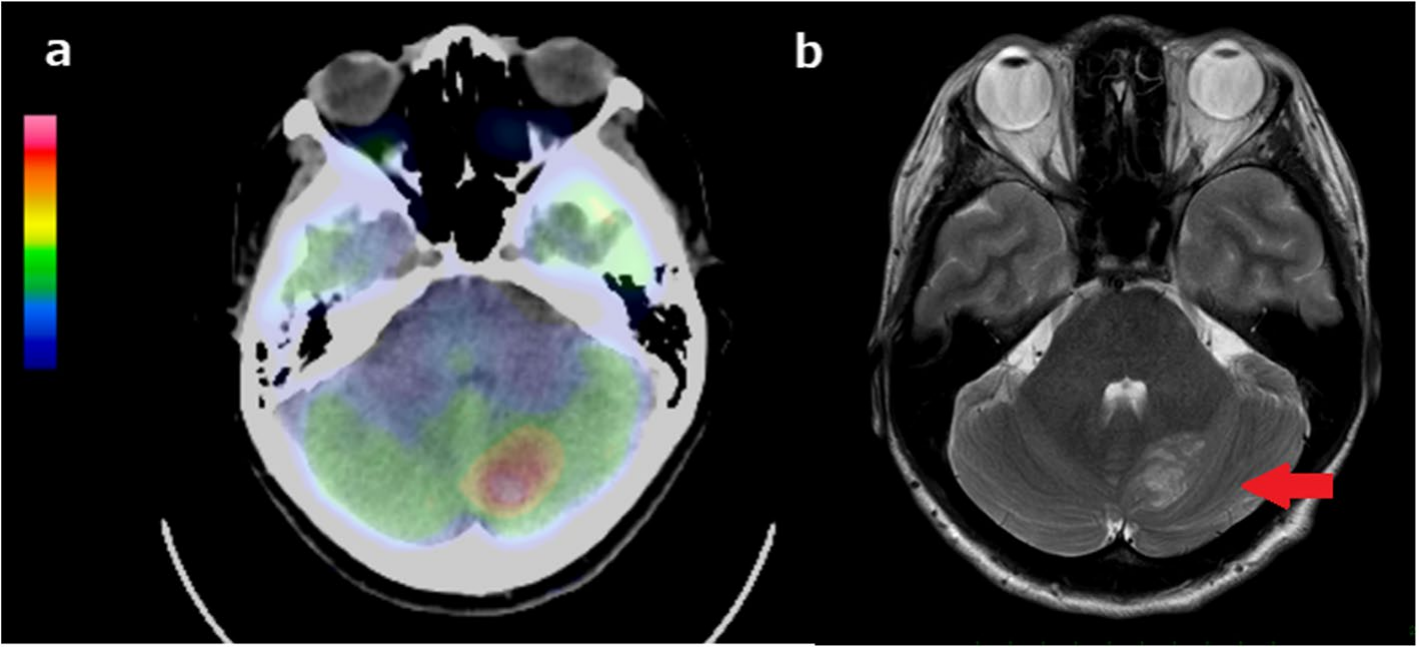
PET-CT demonstrates abnormal uptake with SUV max = 17.8 in the left cerebellar hemisphere (a). T2-weighted MRI reveals hyperintense bands, which suggested “tiger-stripe” appearance (red arrow) (b)
